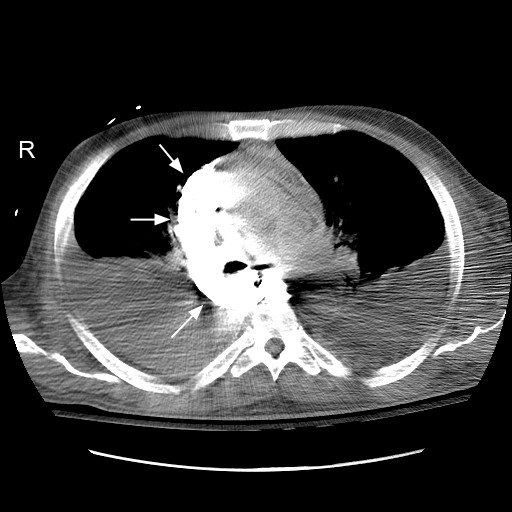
CT scan of chest with arrows showing the contrast in the mediastinum.
